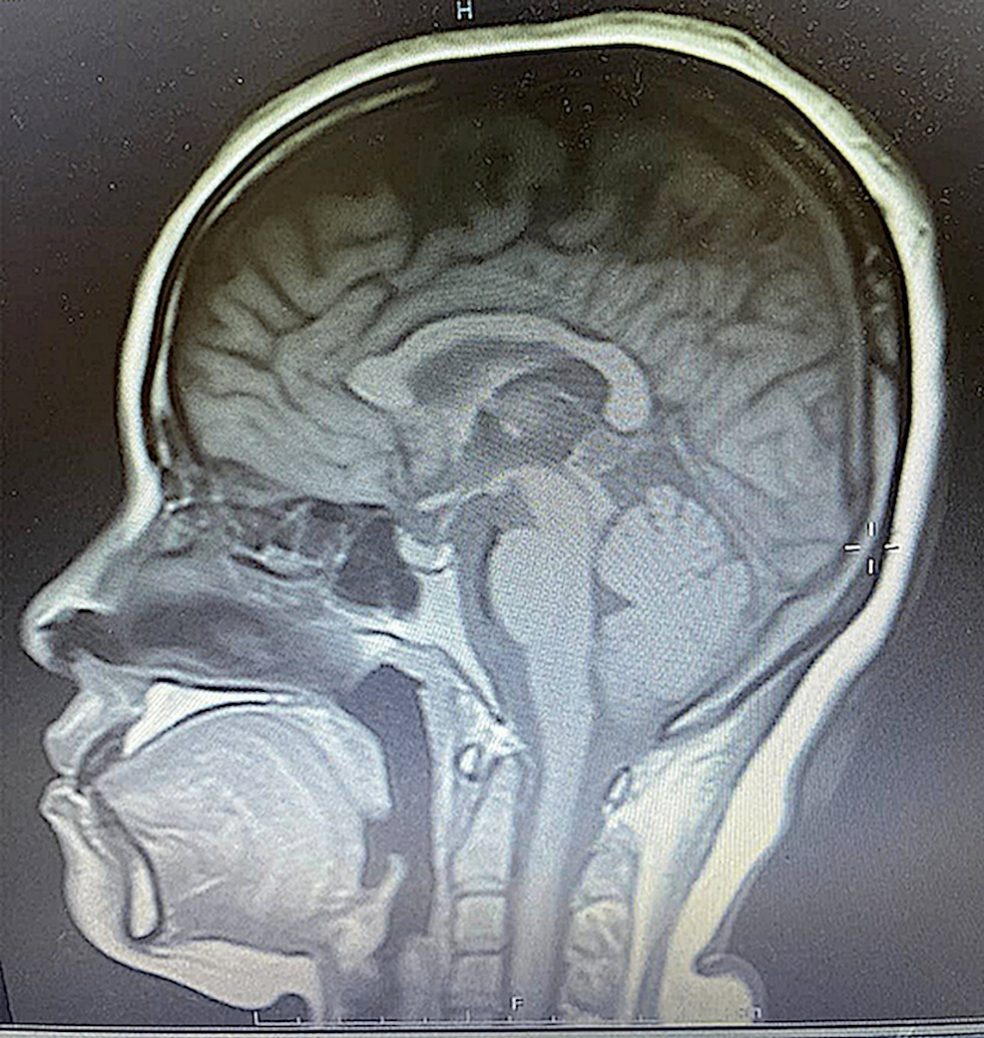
Sagittal view of the brainstem on MRI showing normal findings
